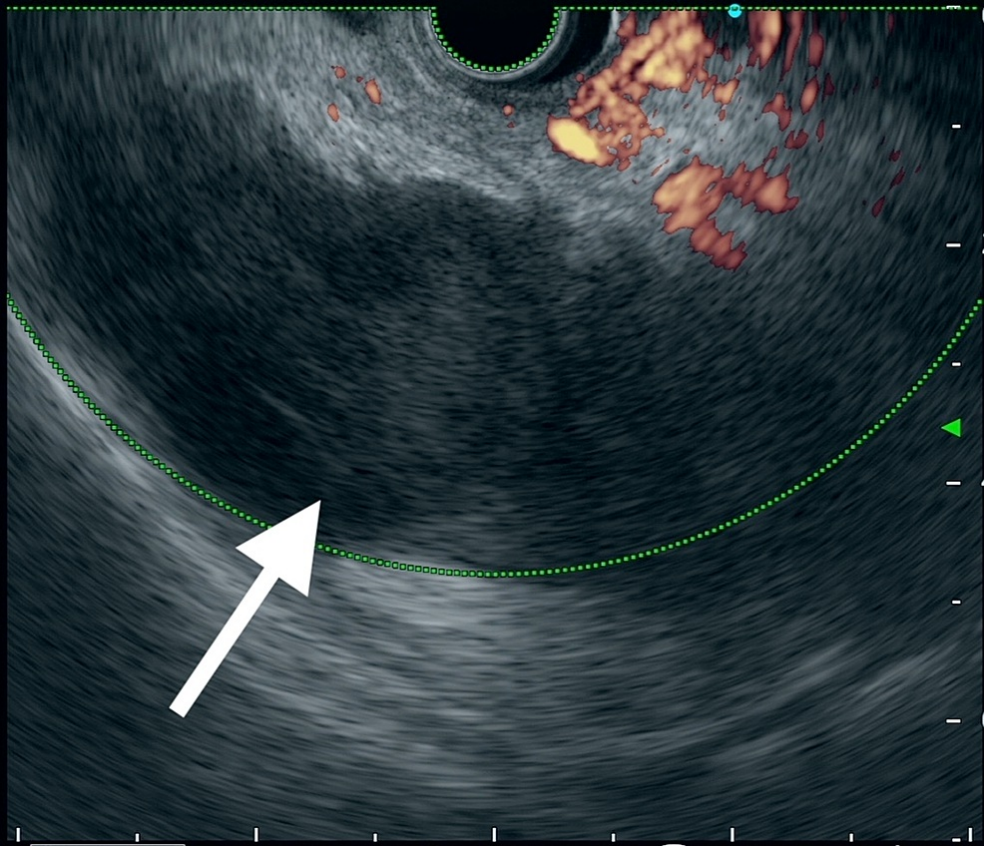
Endoscopic ultrasonography image Endoscopic ultrasonography on three days from admission in Case 2 shows the splenic infarction
